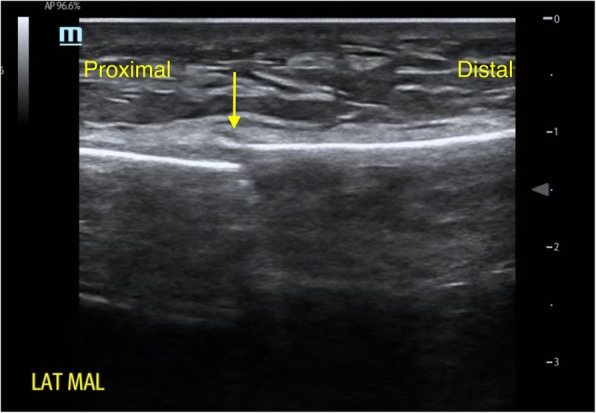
Ultrasound image of the lateral malleolus (= lat mal). The arrow points at cortical disruption
